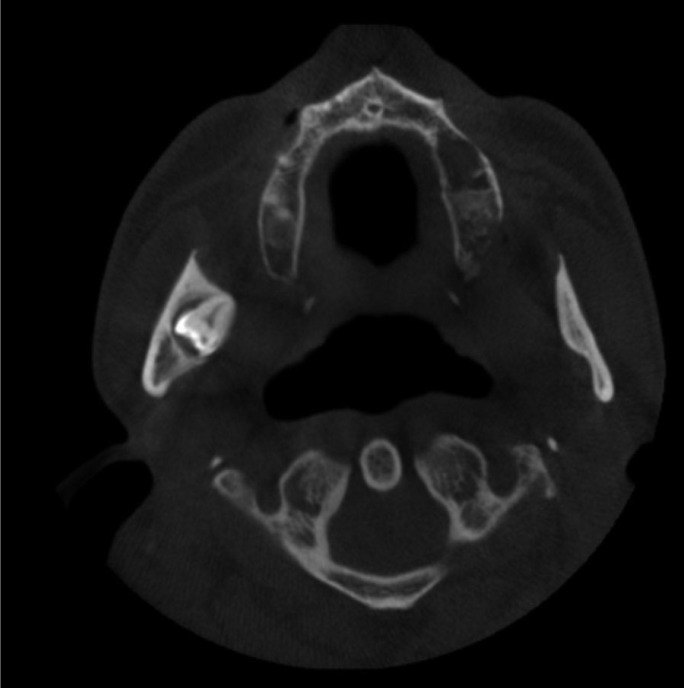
CBCT view of the ectopic third molar.
